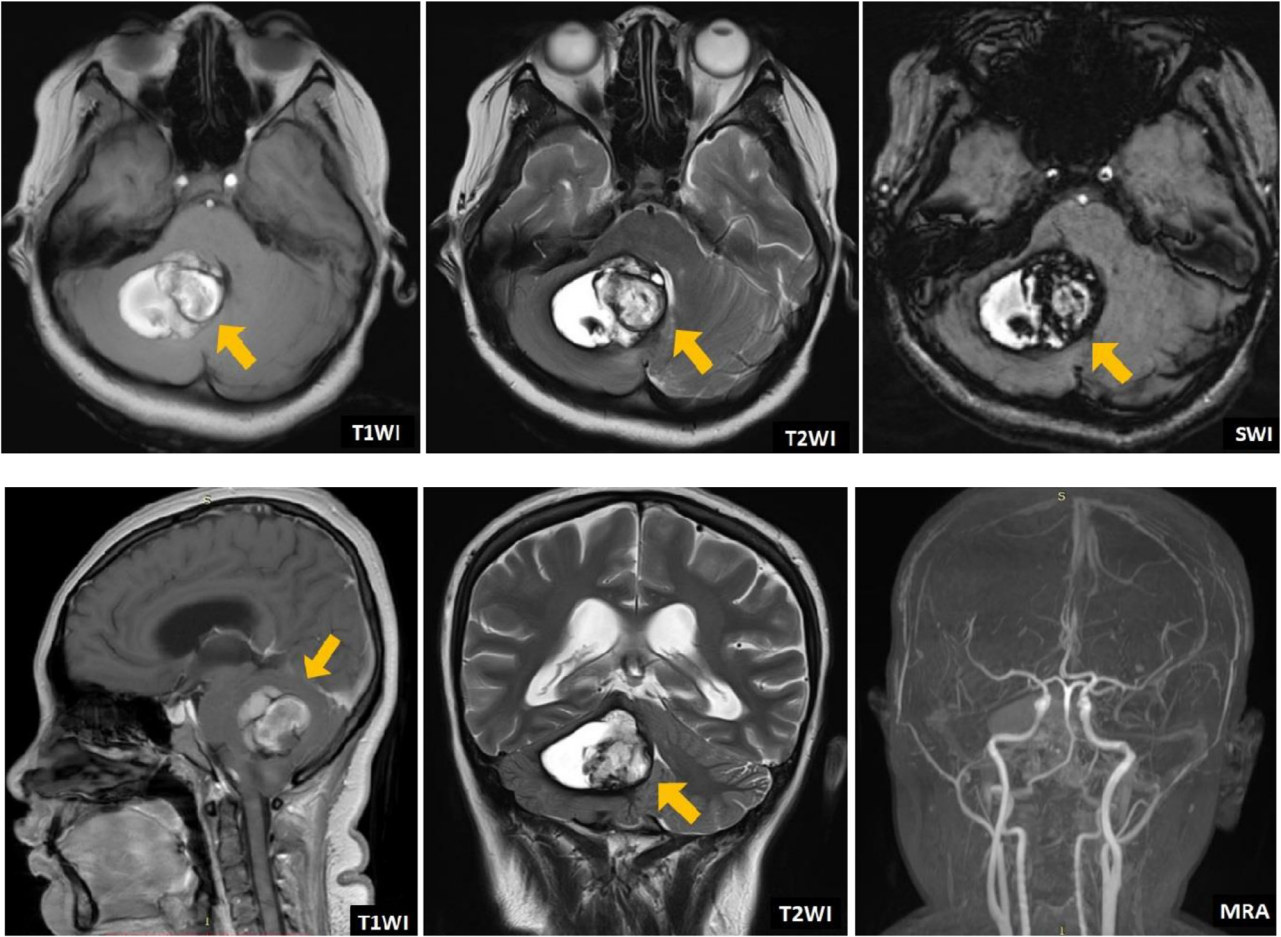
A brain MRI was performed prior to surgery. Yellow arrow in this picture shows the appearance of CCM on axial, coronal and sagittal view. MRA revealed no vascular malformations.
